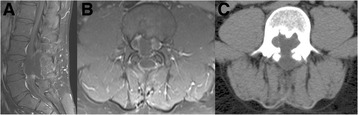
T1-weighted postoperative MR images of the lumbar spine after contrast application showed no tumor recurrence two years after surgery. Sagittal T1-weighted postoperative MR images of the lumbar spine (A). Axial T1-weighted postoperative MR images of the lumbar spine (B, C). MR, magnetic resonance.
